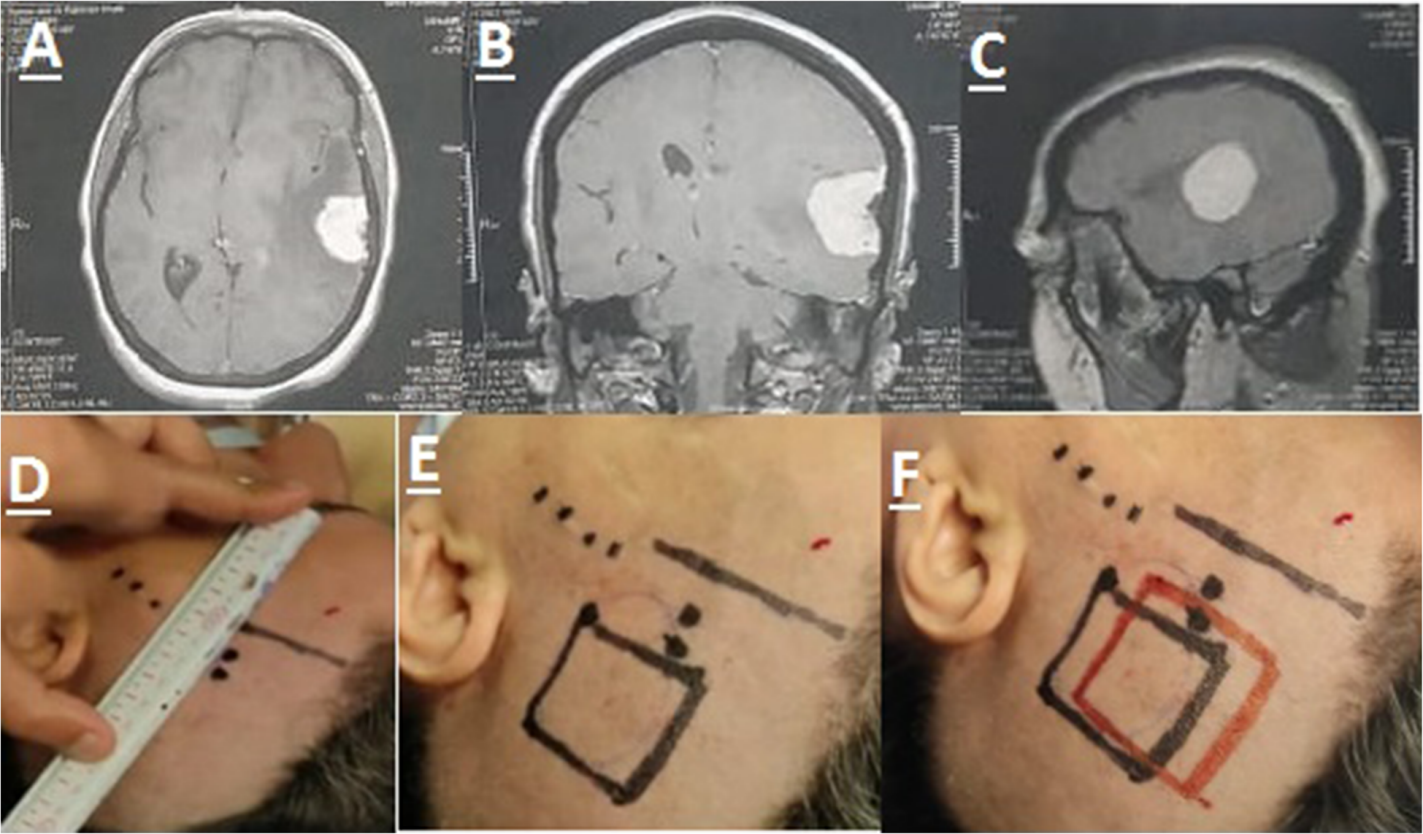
A-C Pictures show contrast MRI study for a convexity meningioma case. D Picture shows the localization of the tumor margins of the tumor using a ruler to calculate the distance from bony landmarks. E Picture shows the localization of the whole tumor using the conventional method marked with the black rectangle. F Picture shows the both methods of localization, and the black rectangle is the craniometric localization, while the red rectangle is the navigation assisted localization
